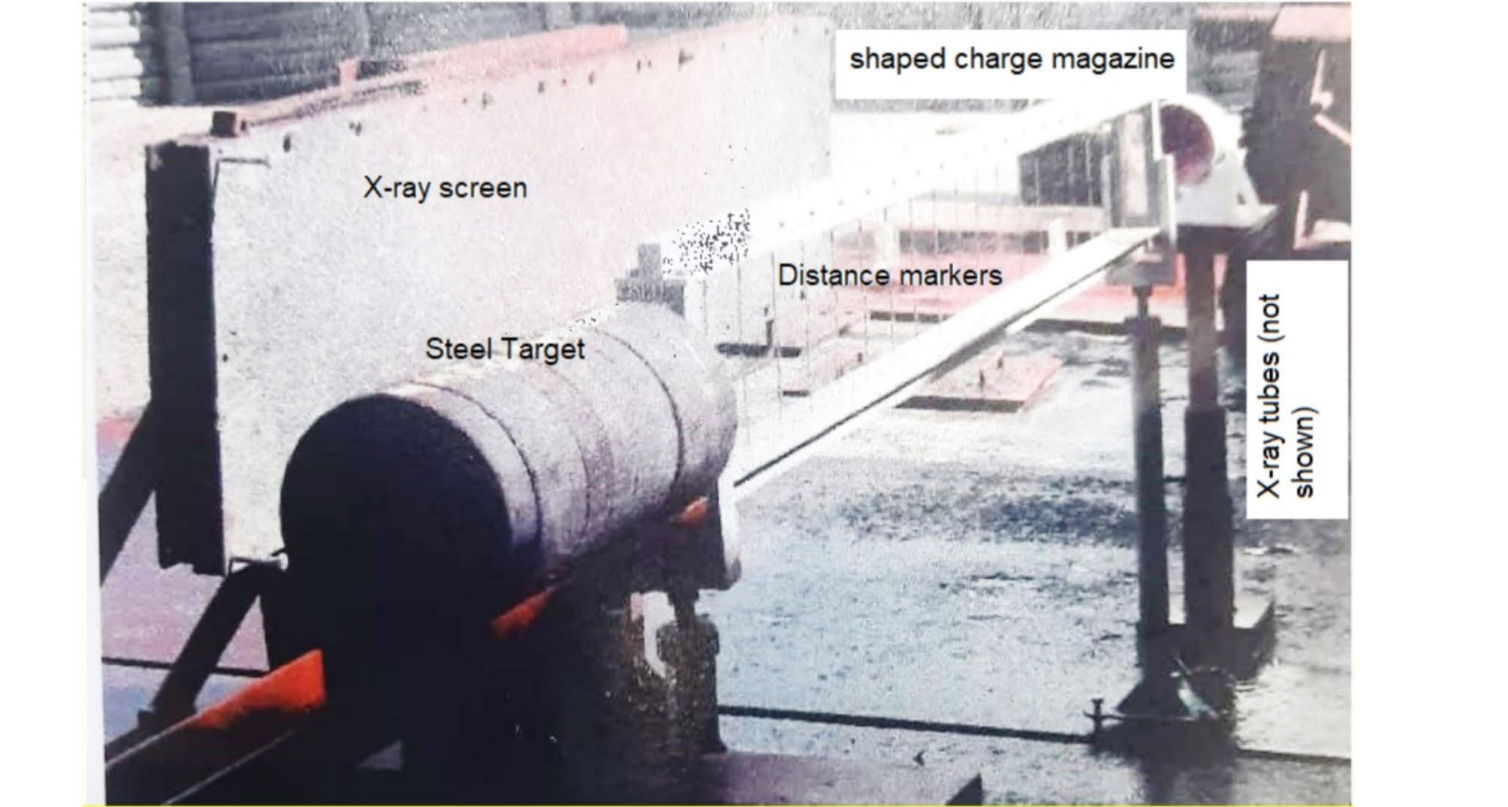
The flash x-ray trial setup.
